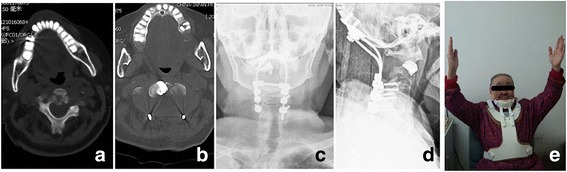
A 78-year-old female with a C2 metastatic tumour, presenting with occipitocervical pain and quadriplegia, was treated with intraoperative vertebroplasty combined with posterior decompression and occipitocervical fixation in 2012. a and b Preoperative and postoperative axial CT scans. c and d Postoperative anterior, posterior and lateral radiographs. e The muscle power of the patient returned to normal and pain was completely relieved
